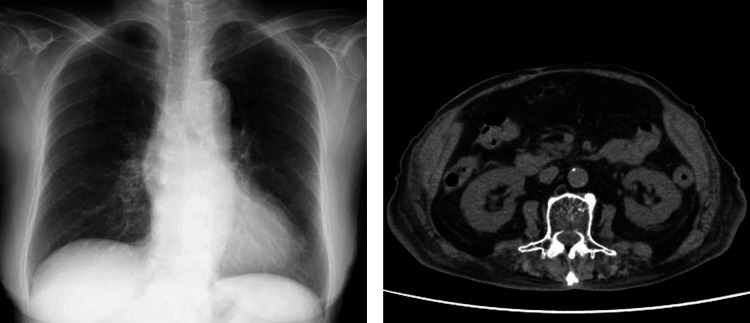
Chest X-ray and abdominal computed tomography before the initiation of shichimotsukokato
